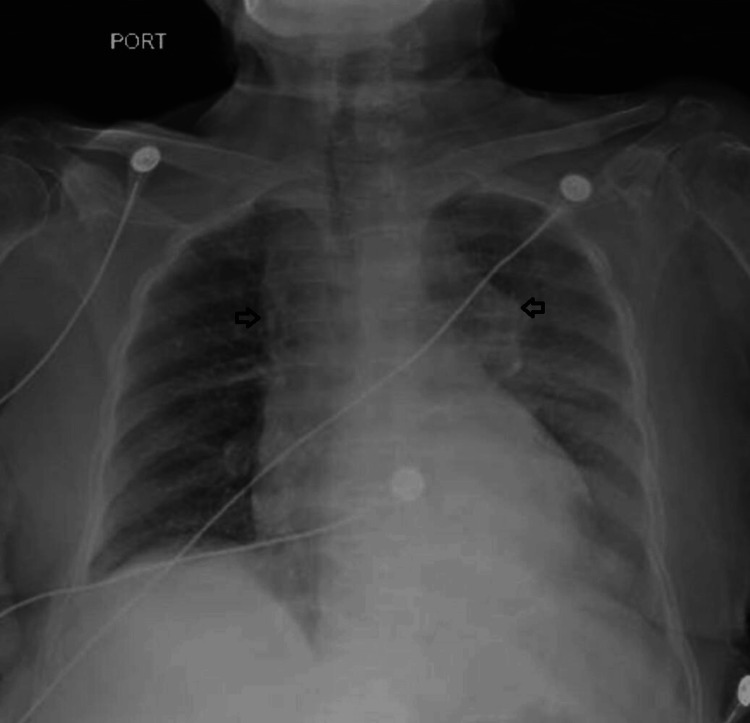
Initial anteroposterior chest X-ray displaying mediastinal widening (black arrows)
